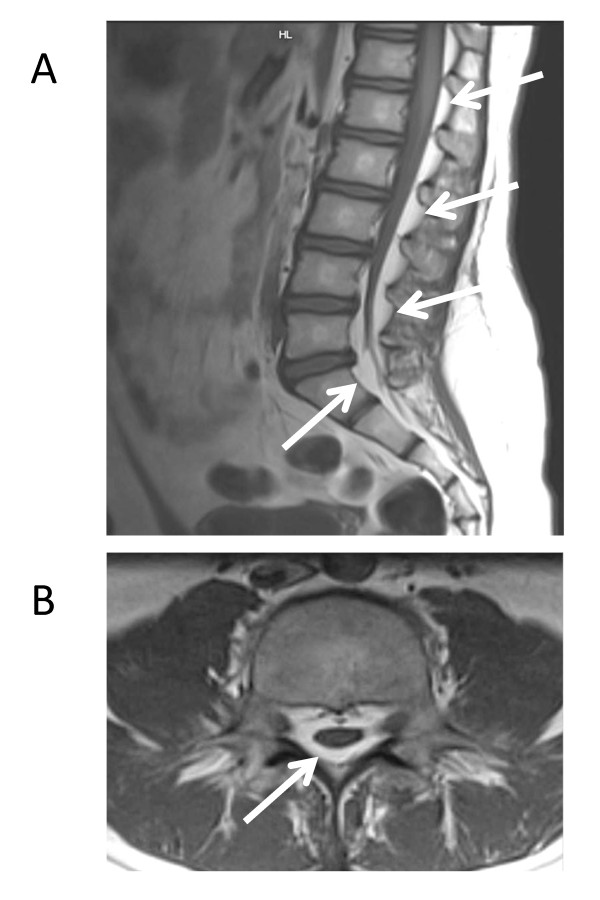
MRI including the lumbar spine, demonstrating spinal epidural lipomatosis in the presented patient after 4.5 months of treatment with prednisone and methylprednisolone. The arrows point to selected areas of the pathological accumulation of fatty tissue in the epidural space. This was not present in a baseline MRI performed before initiation of corticosteroid therapy (image not shown). T1 weighted images; A, sagittal plane; B, axial plane.
